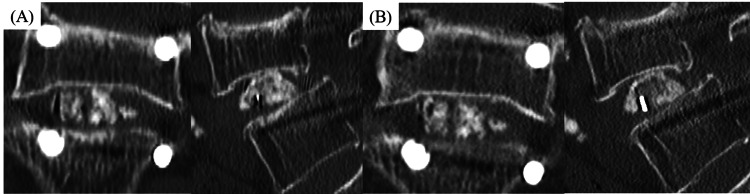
Representative case 1 (A) Case 1 with translucent zone comprising more than 50% of vertebral diameter between autograft and endplate two months postoperatively. Coronal CT slice in the center cage region was evaluated as grade II, sagittal CT slice in the central cage region was evaluated as grade I (for a total score of 3). (B) Case 1 without complete osseous union six months postoperatively. Coronal CT slice in the central cage region was evaluated as grade III, sagittal CT slice in the central cage region was evaluated as grade II (for a total score of 5).
